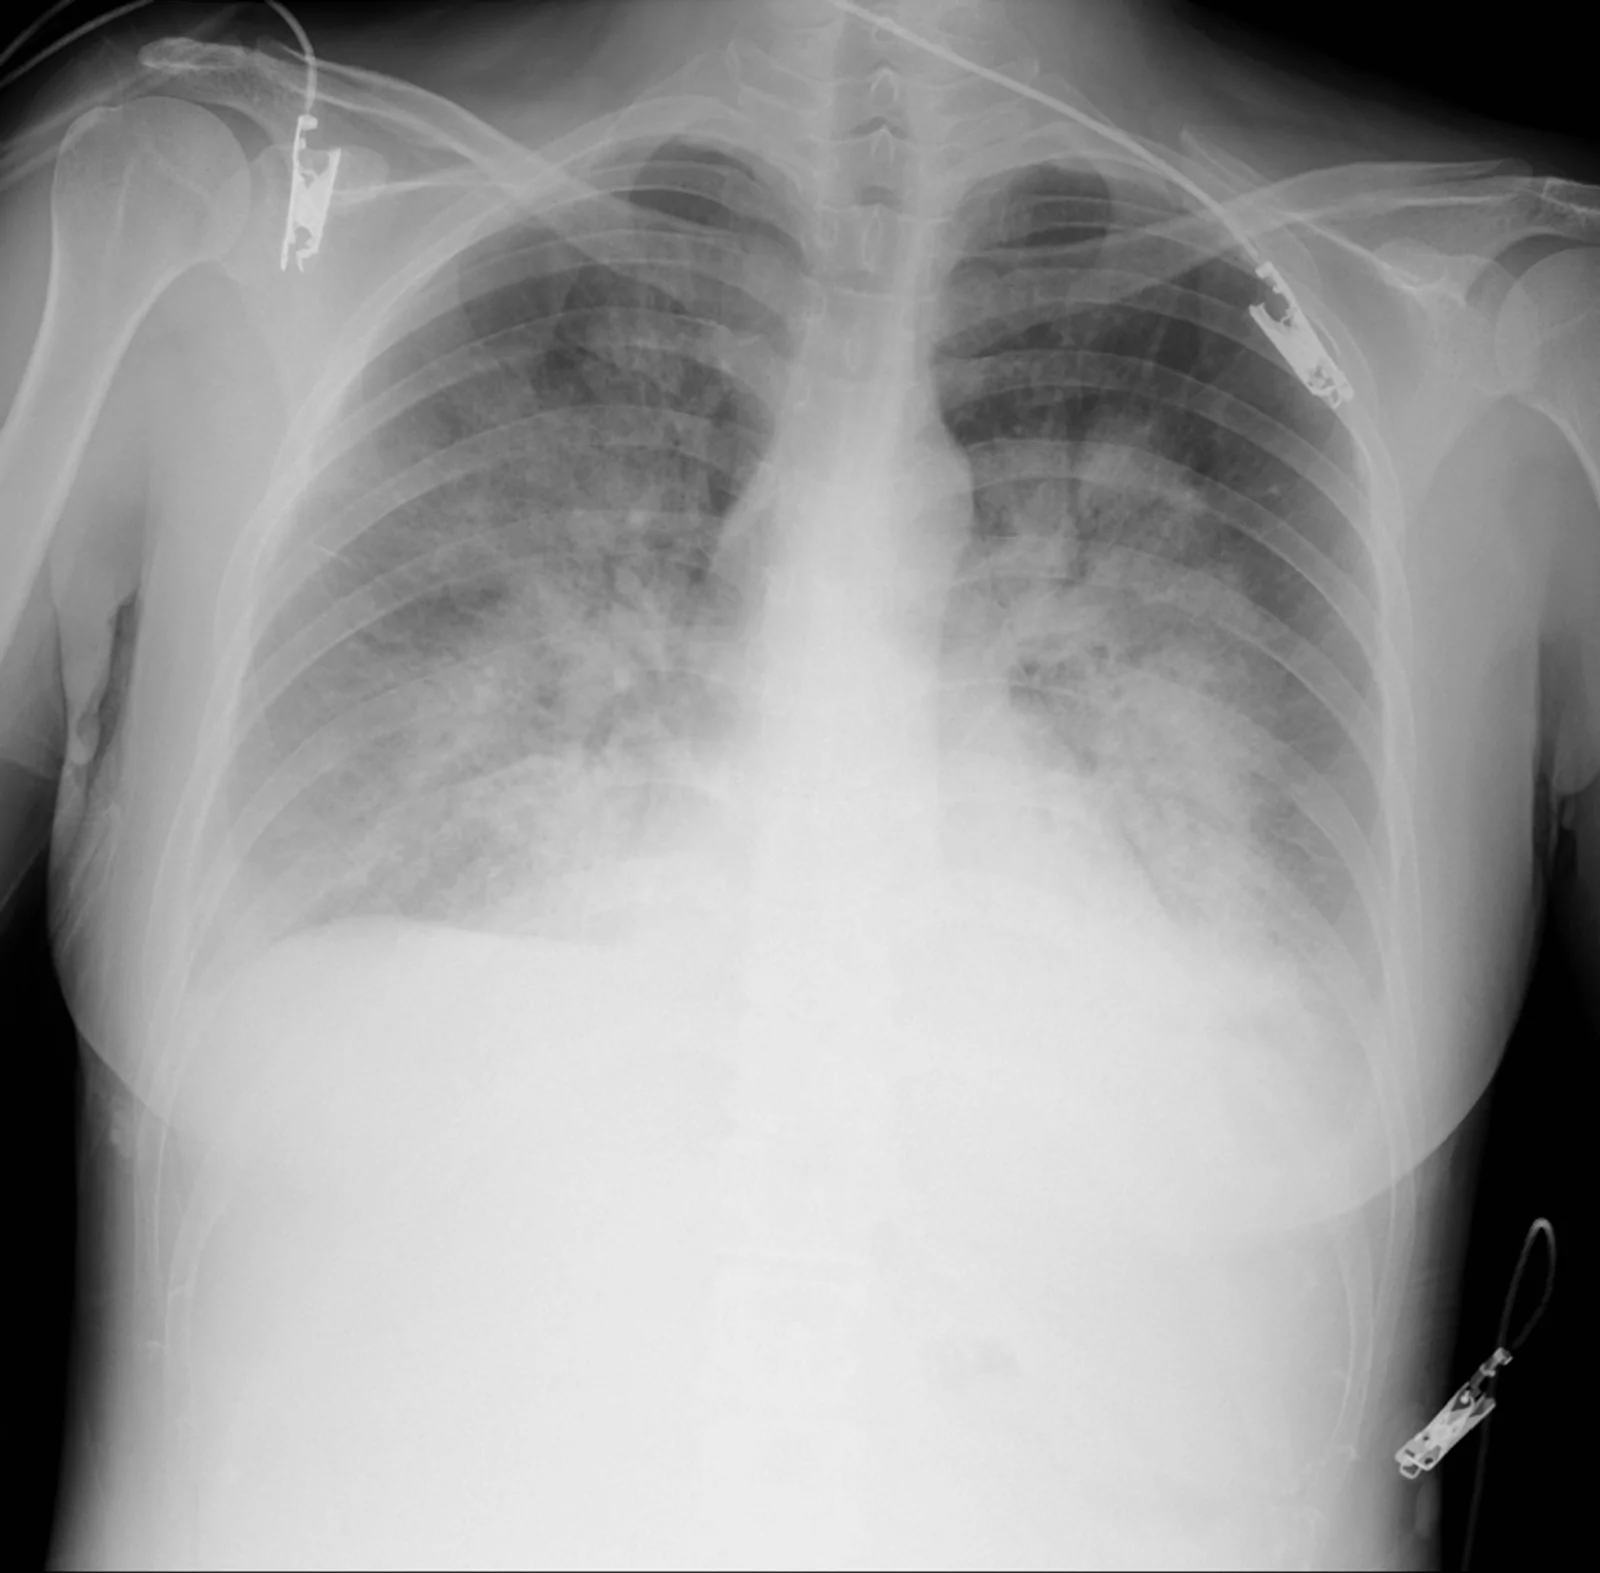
Chest radiograph on admission Chest radiography demonstrated cardiomegaly with a cardiothoracic ratio of 52% and pulmonary vascular congestion.
